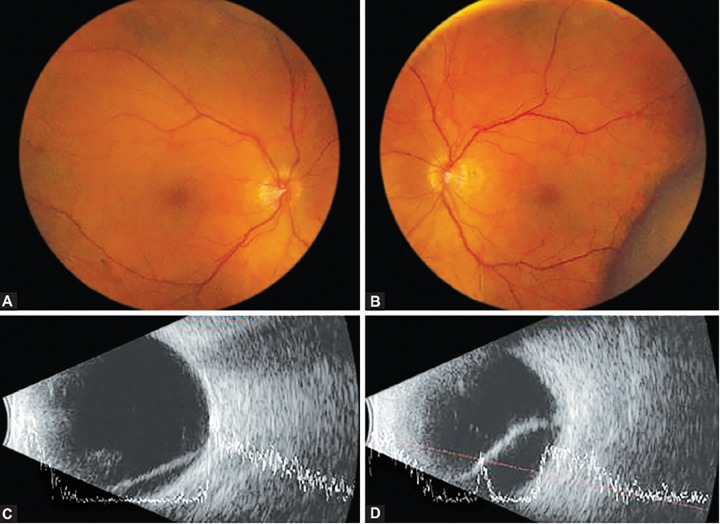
Fundus (A and B) and ultrasound (C and D) images at presentation, revealing a bilateral choroidal effusion
